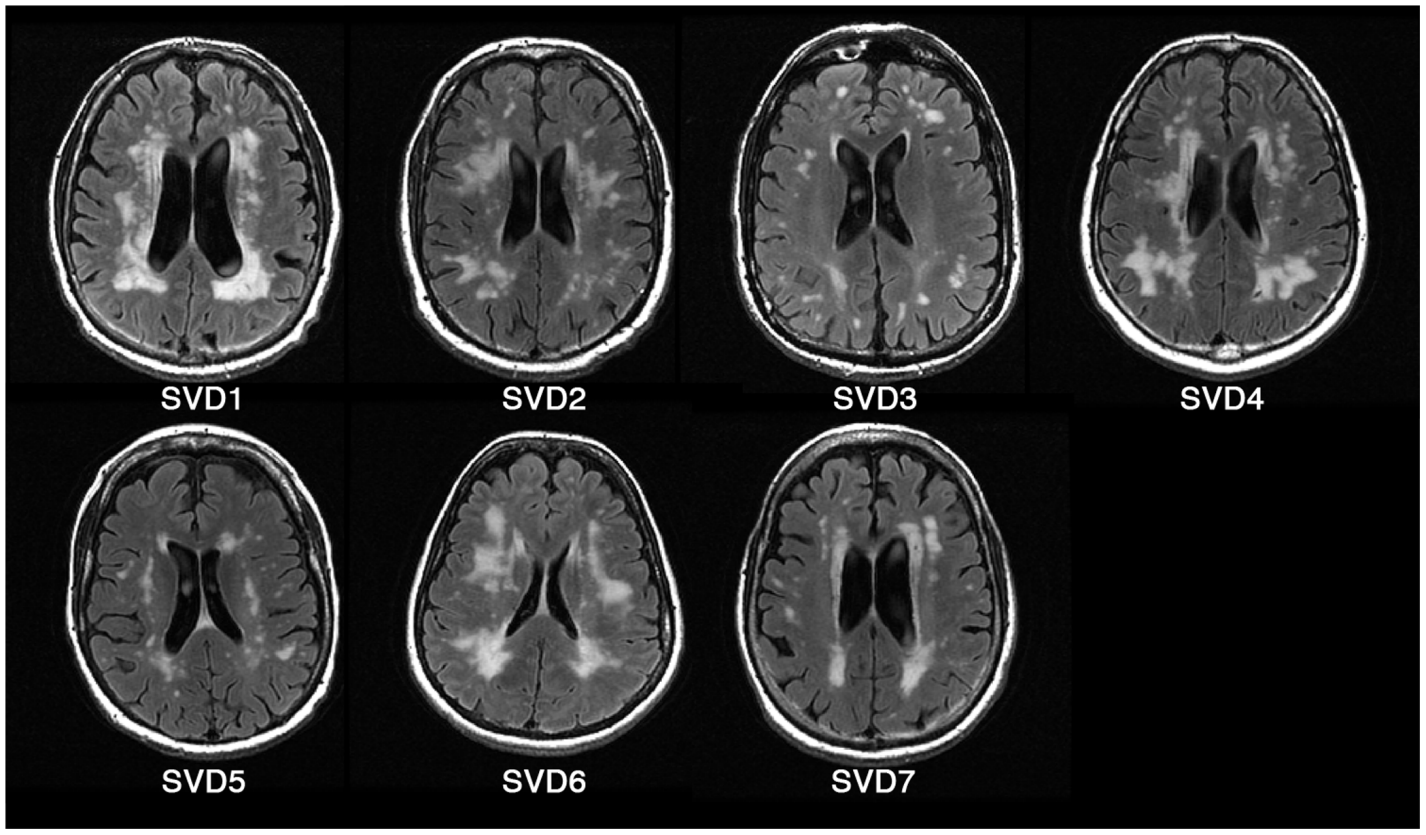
Fluid attenuated inversion recovery (FLAIR) images of SVD participants.
